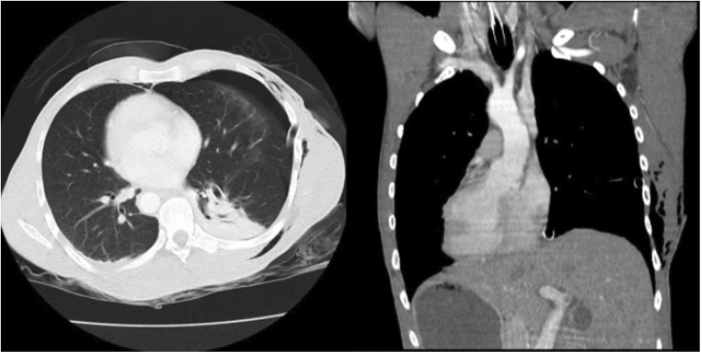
CT scan findings. A - Chest CT scan showing a hemopneumothorax to the left; B - Chest CT scan findings with dextrocardia and mirror-image transposition of the organs in the abdomen, confirming Situs inversus totalis.
